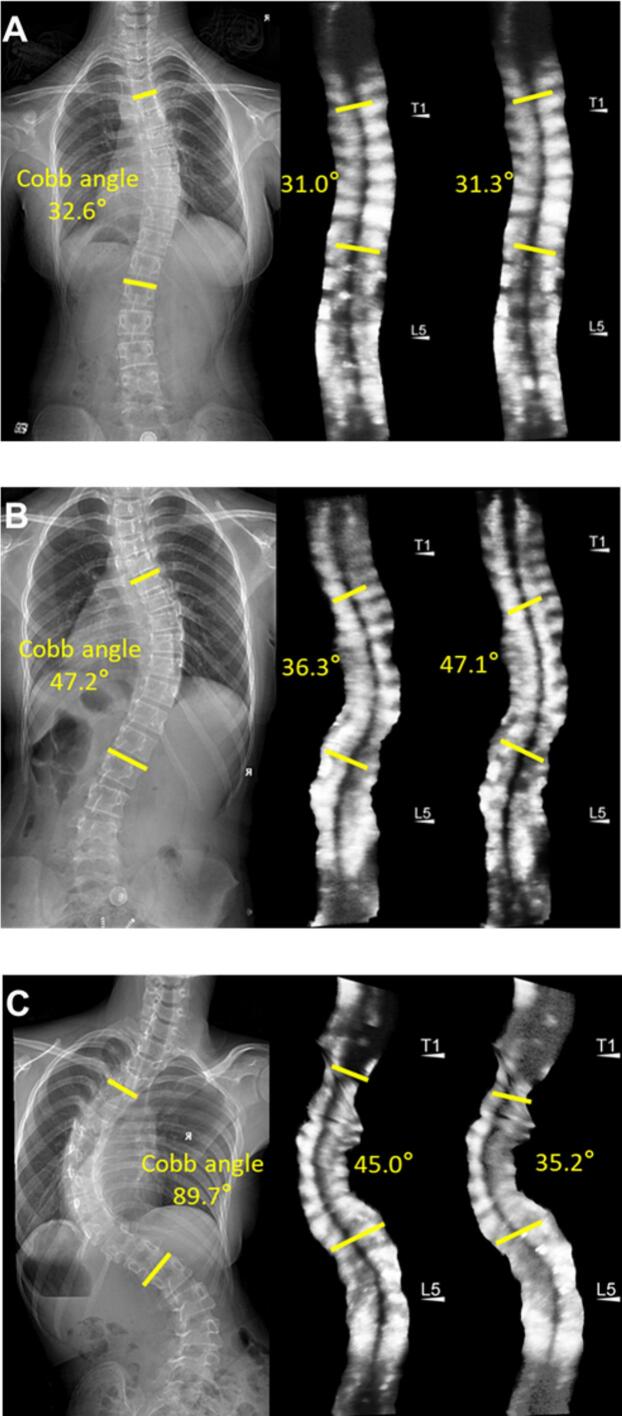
Summary of ultrasound imaging characteristics in AIS. (A) Successful initial and secondary scans. (B) Initial scan suboptimal, secondary scan successful. (C) Persistent imaging challenges despite multiple scanning attempts.
